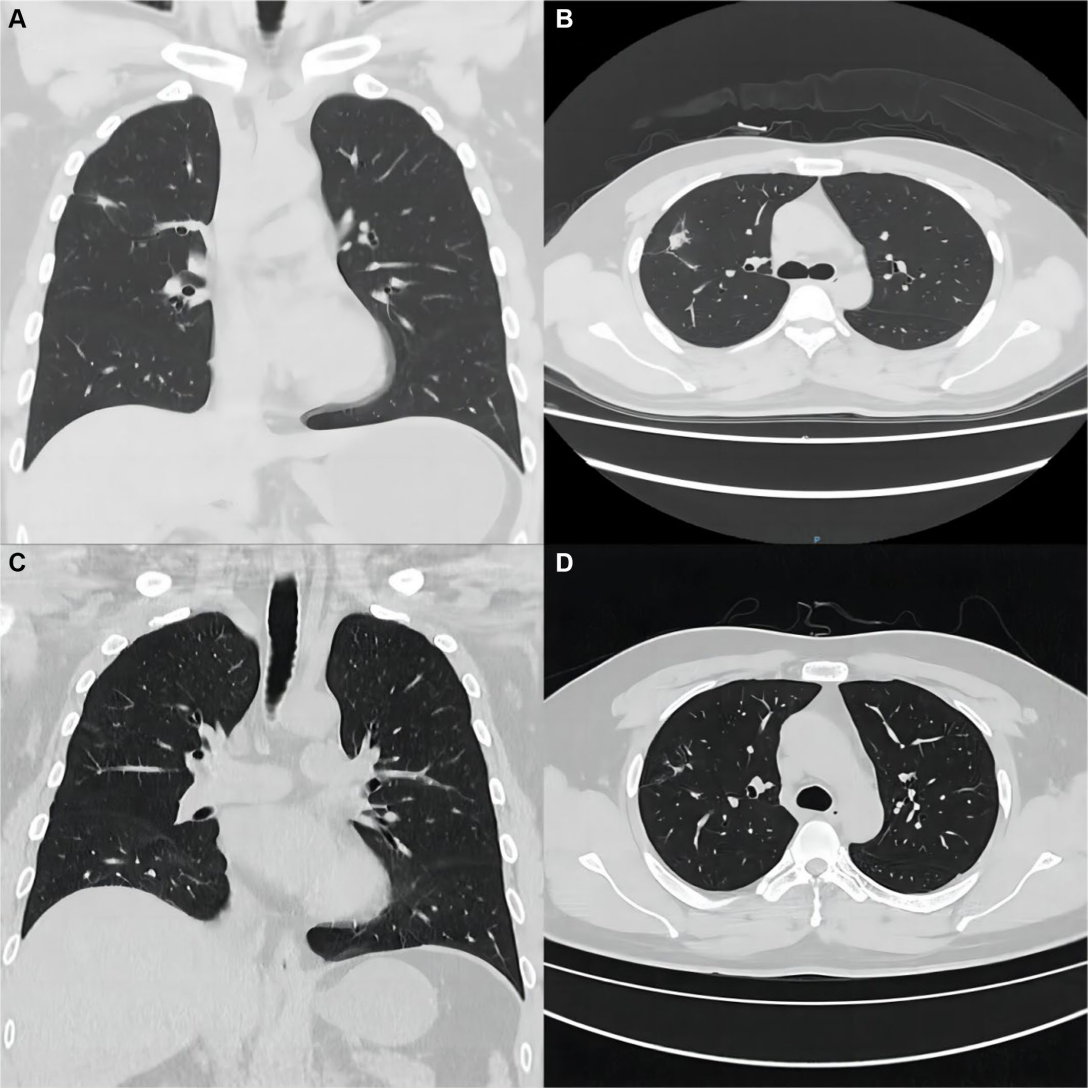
Imaging and pathological findings of the patient at the time of return visit. (A,B) After 6 months of treatment, a repeat chest CT showed significant improvement of the original lung lesion. (C,D) CT scan of the chest at follow-up until May 2022, with only a few fibrous lesions remaining from the original lung lesion.
